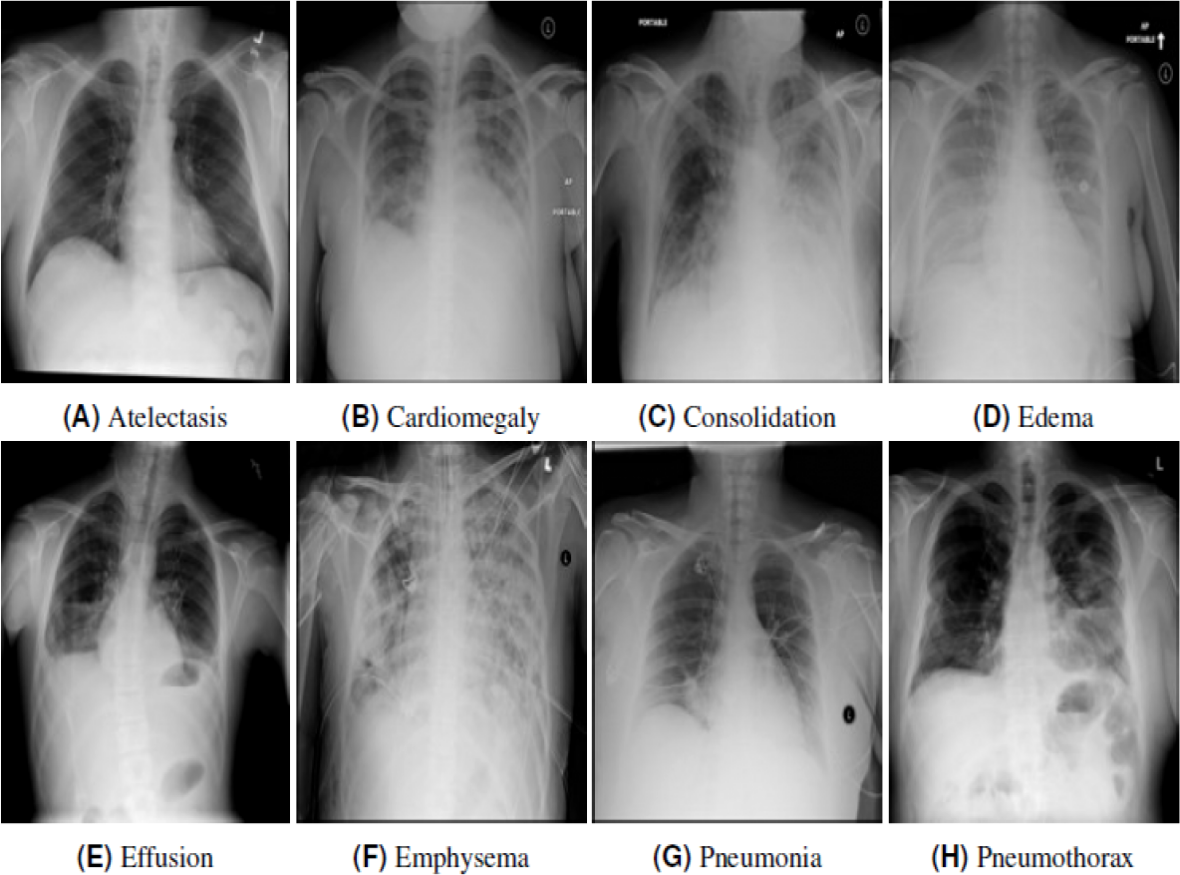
Chest X-rays categories pathology with related labels in Chest X-ray14. Each image is labelled with one pathology. (A) Atelectasis, (B) cardiomegaly, (C) consolidation, (D) edema, (E) effusion, (F) emphysema, (G) pneumonia, (H) phenothorax.
